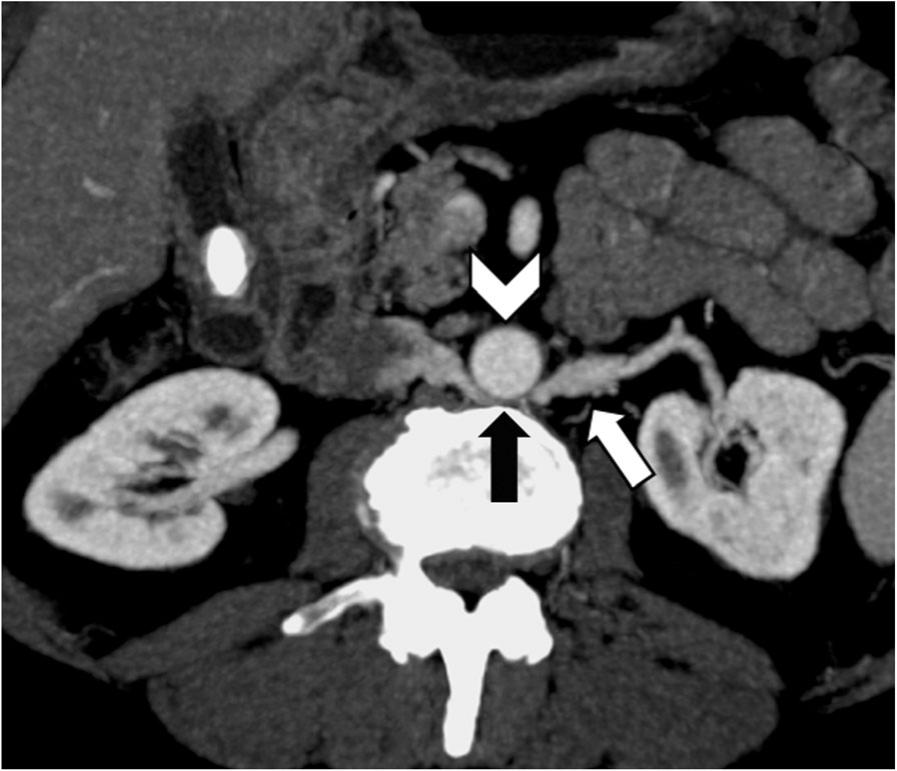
A 78-year-old man with posterior nutcracker syndrome. Axial CT image on the arterial phase shows left renal vein (white arrow) compressed between the aorta (arrowhead) and the vertebral body (black arrow)
